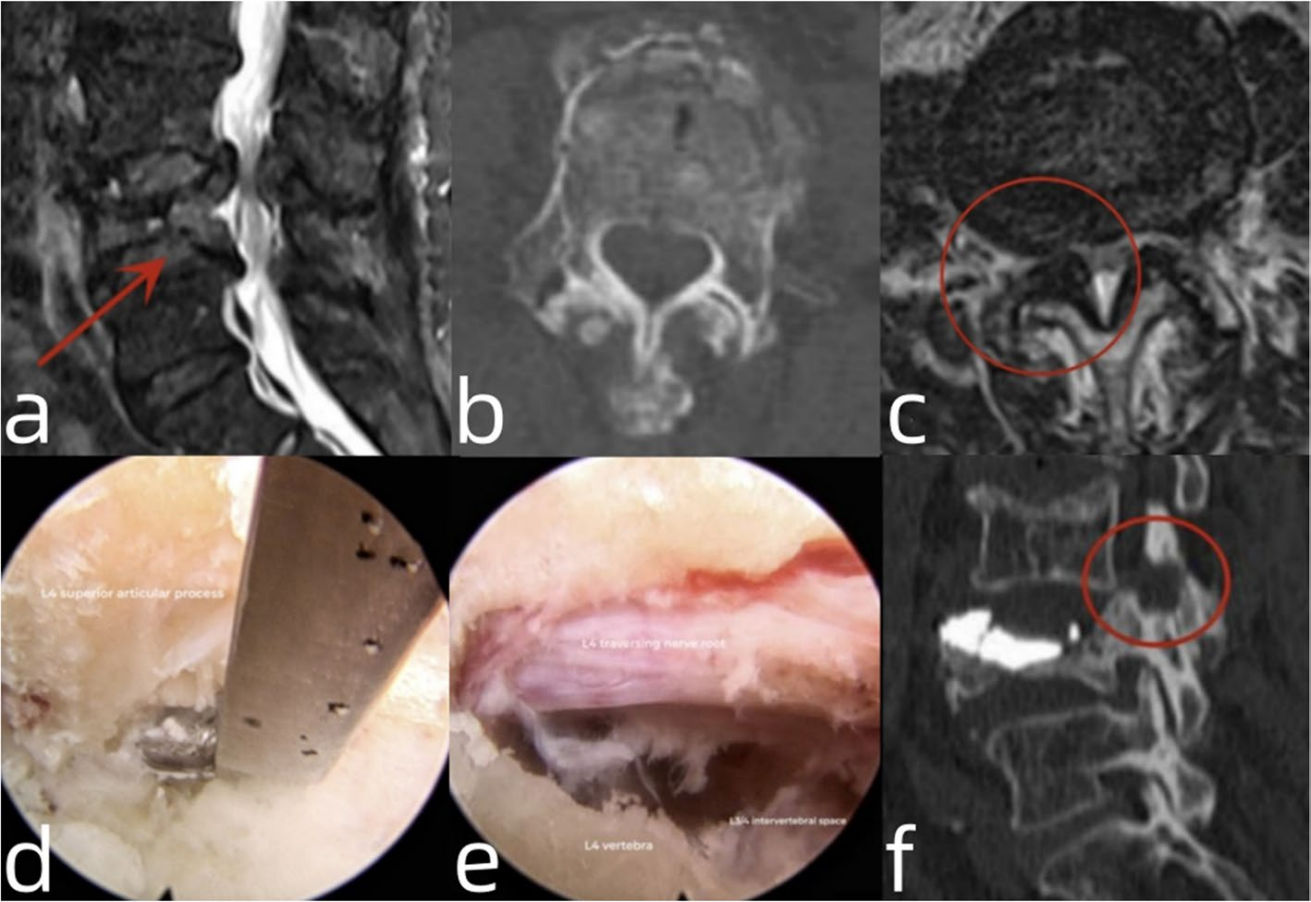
a Sagittal view of T2-weighted MRI revealing the fracture of L4 vertebra; b: Cross-sectional CT showing fracture of L4 IEP; c: Cross-sectional MRI showing stenosis of the right L3/4 foramen; d: Foraminoplasty on L3/4 with a Kerrison rongeur under full-endoscopic view; e: The L4 traversing nerve root was completely decompressed; f: Postoperative sagittal CT showing a partial resection of L4 superior articular process and pedicle, with no cement leakage in the foramen
